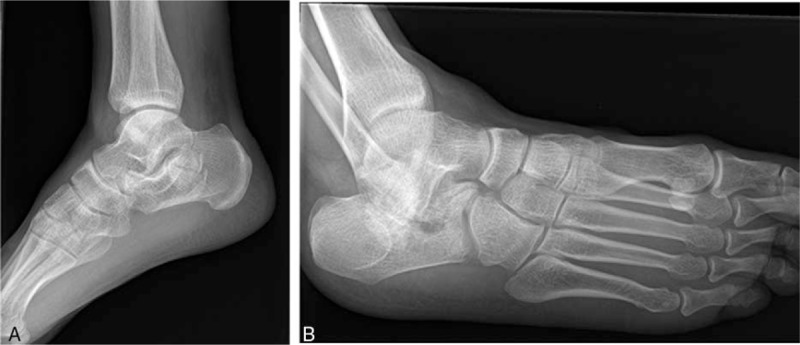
(A) The X-ray showed bony irregularity and a higher density region on the corpus calcaneus. (B) The bone defect was found in the forepart of the calcaneus by oblique radiograph.
